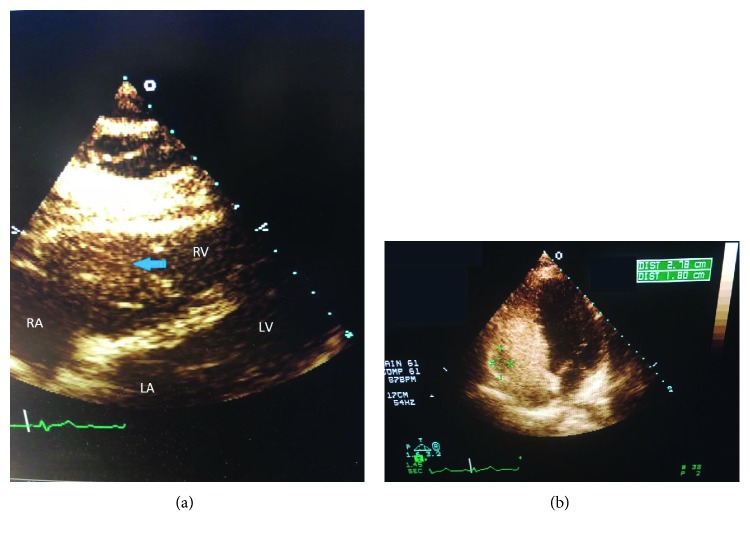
(a) Transthoracic echocardiogram with right ventricular myxoma (arrow). (b) Transthoracic echocardiogram with agitated saline revealing a right ventricular myxoma measuring 2.78 cm × 1.8 cm.
